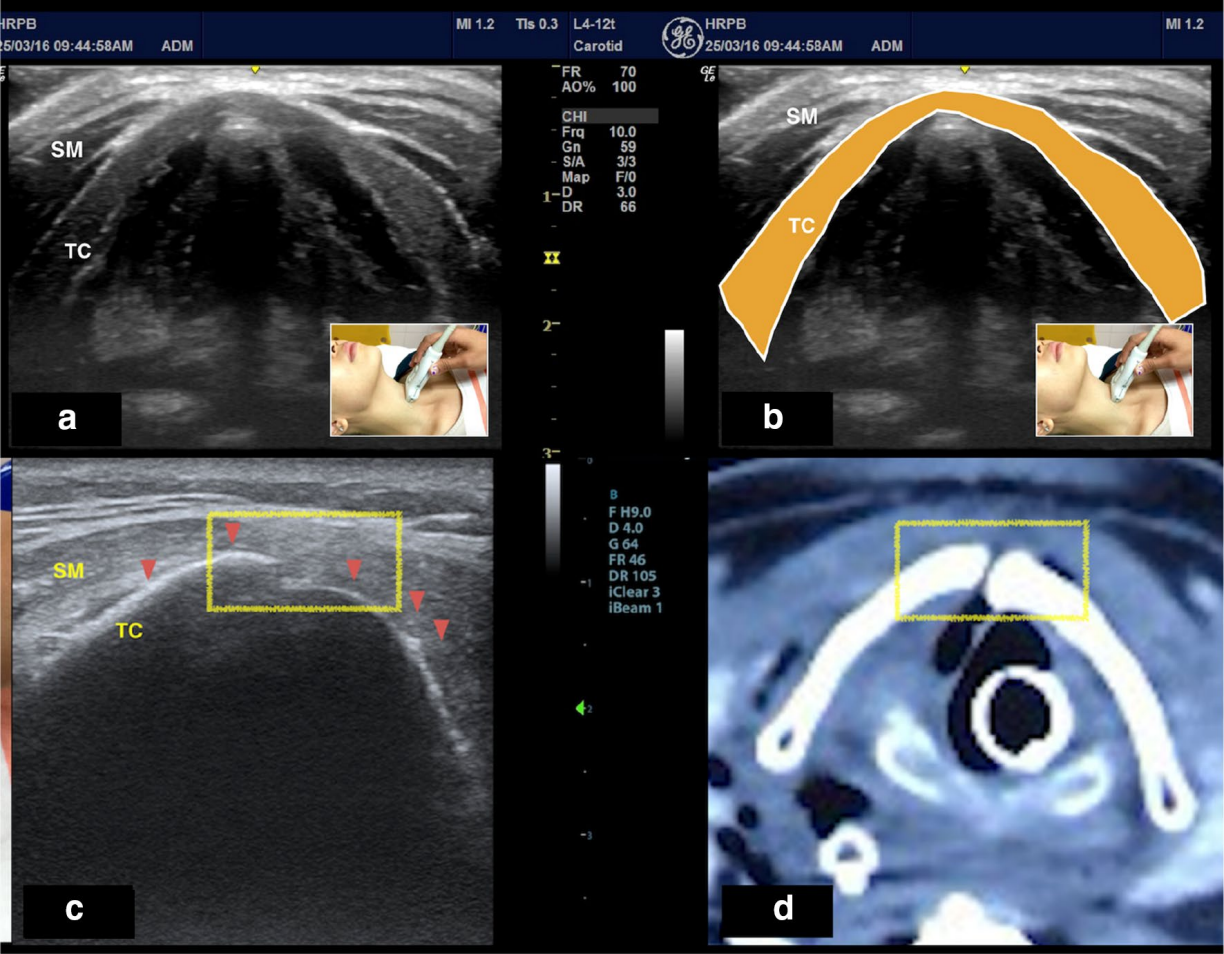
a, b Surface landmark of the probe position and ultrasound image of the normal thyroid cartilage (in transverse view). c Airway ultrasound showing undisplaced fracture of the thyroid cartilage (box) and disruption of the anterior cortex of the thyroid cartilage (arrowhead). d A Computerized tomography (CT) scan image shown right thyroid lamina fracture with surrounding prevertebral edema and hematoma. SM: sternocleidomastoid muscle; TC: thyroid cartilage
